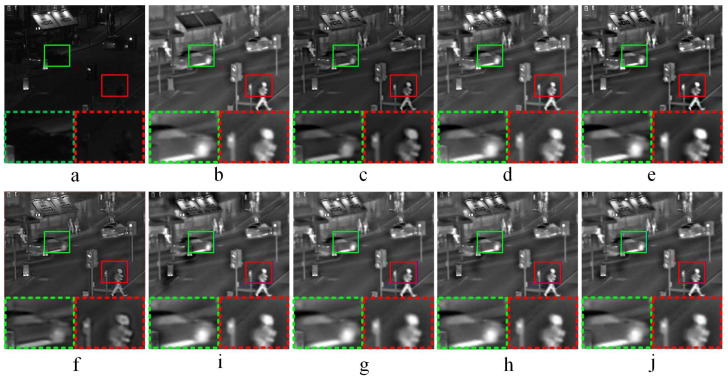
Infrared-visible image fusion comparative experiments—1. (a,b) are source images, (c–j) are the fused results of ASR, CNN, CT, KIM, MST-SR, NSCT-PCNN, NSCT-PC, and the proposed method, respectively. At the bottom of each image, two areas marked in green and red dashed line frames correspond to the magnified areas encompassed in green and red frames, respectively.
